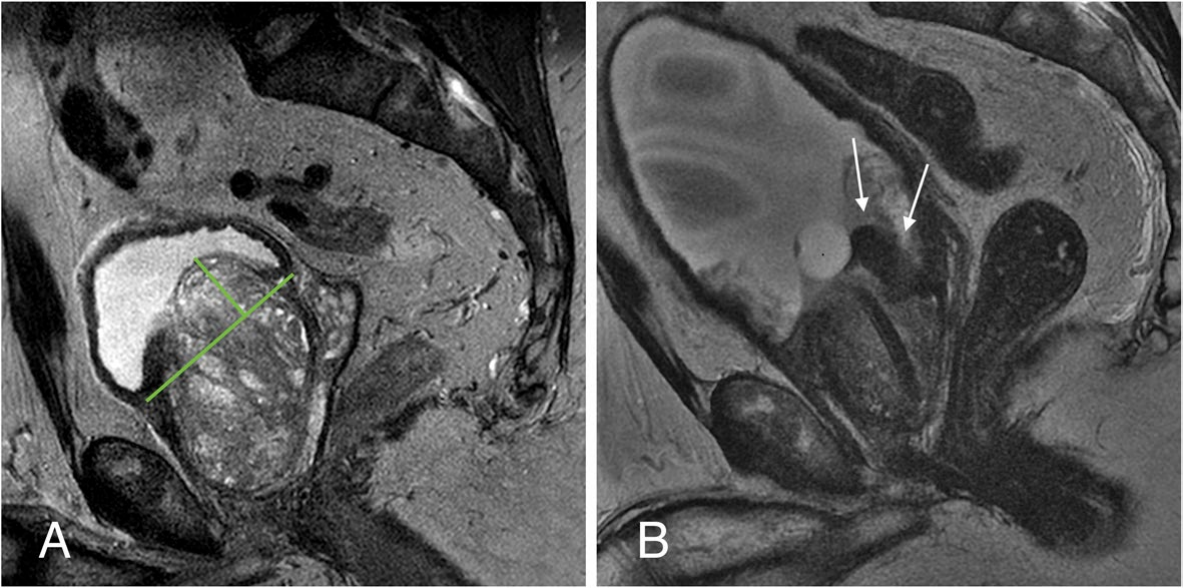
A Pre PAE T2-weighted MRI in sagittal view showing a very enlarged prostate, with a large medium lobe (grade III IPP = 26 mm). B After embolization, marked hypointense signal and volumetric reduction of the medium lobe was observed (arrows). (*) Foley balloon. A cystoscopy confirmed the presence of a necrotic medium lobe leading to ball-valve effect
